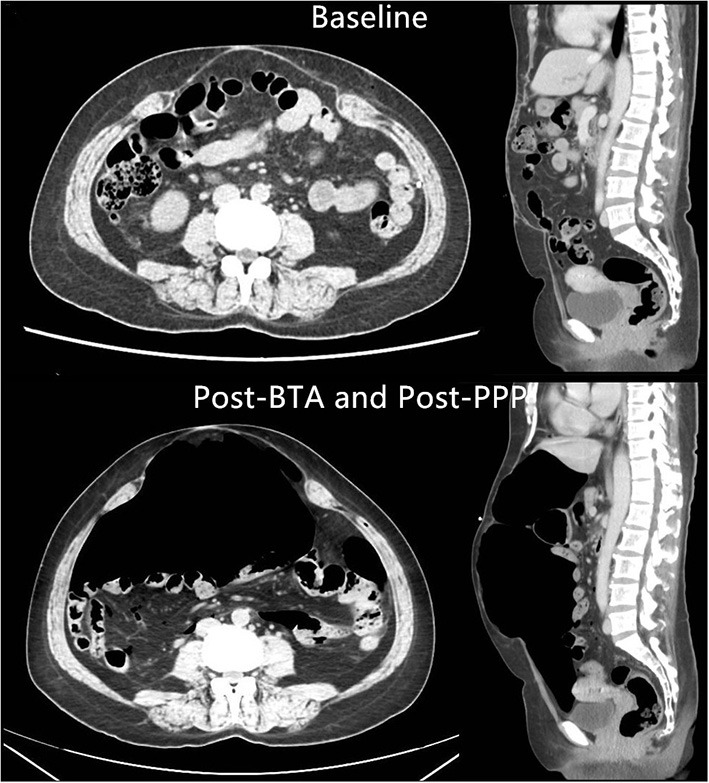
Abdominal CT image comparison of pre-and post-PPP treatments demonstrating spontaneously reinstate the herniated contents (PT-2).
